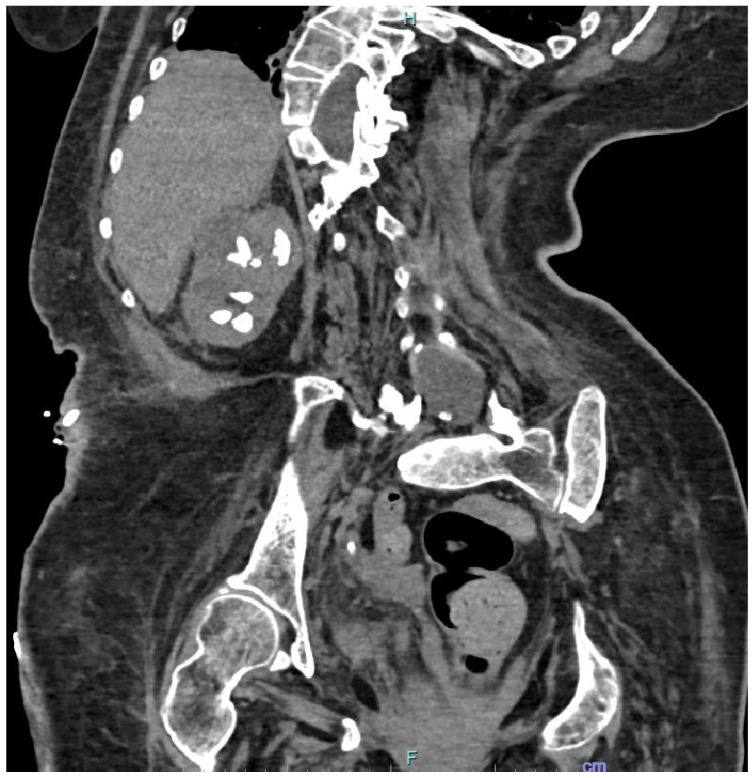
CT scan showing multiple kidney stones in a patient affected by spina bifida.
